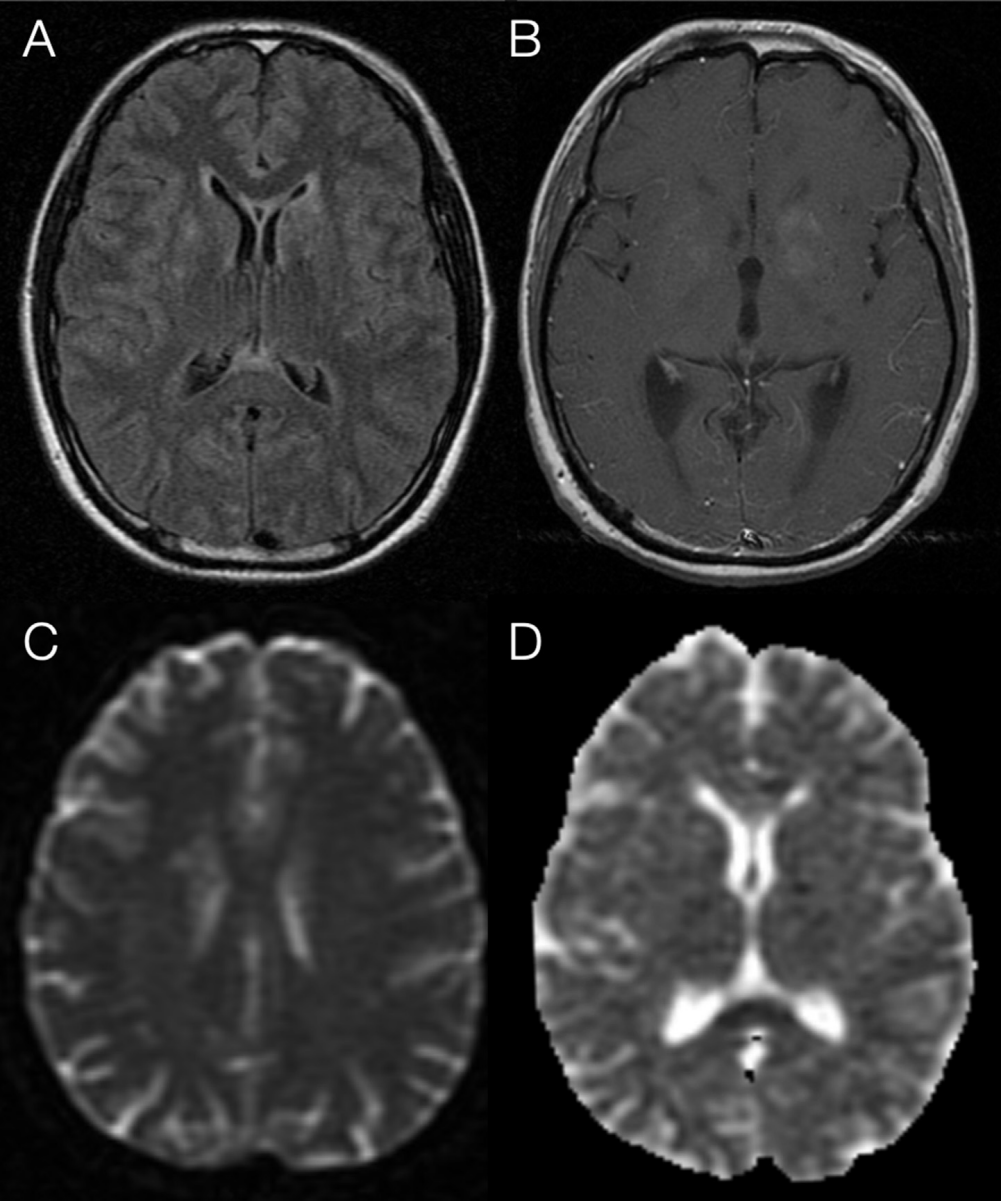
A, B: Brain MR imaging showing basal ganglia T2 hyperintense (A) and T1 contrast-enhancing areas (B), suggestive of cryptococcal lesions. C, D: Subcortical white matter diffusion weighted imaging (DWI) hyperintensities, possibly related to small vessel vasculitic phenomena. D: Apparent diffusion coefficient (ADC) map showing hypointensity in the splenium of corpus callosum, as previously described in cases of cryptococcal encephalitis.
